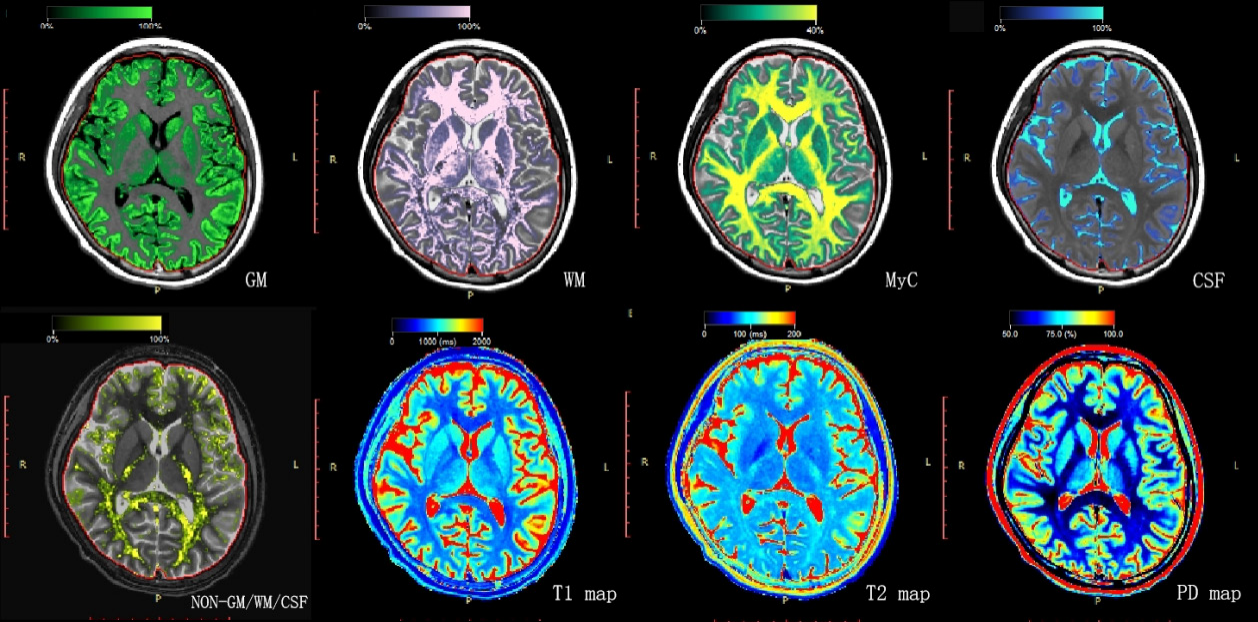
The grey matter volume, white matter volume, myelin content, cerebrospinal fluid volume and quantitative map of T1, T2 and PD were obtained by synthetic MRI (SyMRI). CSF, cerebrospinal fluid; GM, grey matter; MyC, myelin content; PD, proton density; T1, longitudinal relaxation time; T2, transverse relaxation time; WM, white matter.
